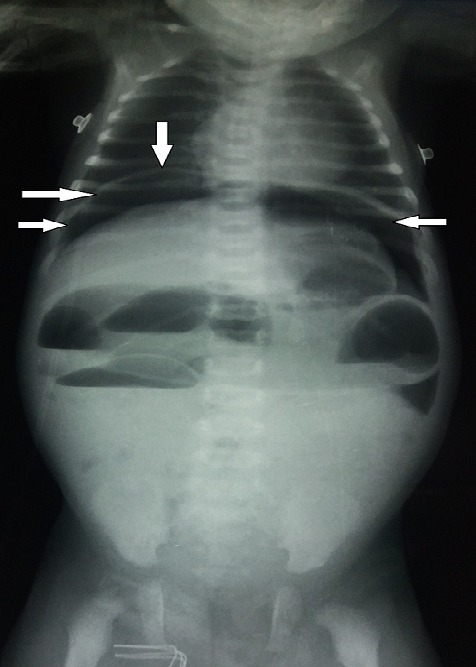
radiographie thoraco-abdominale montrant plusieurs niveaux hydro-aériques sans aération rectale avec un pneumopéritoine massif
